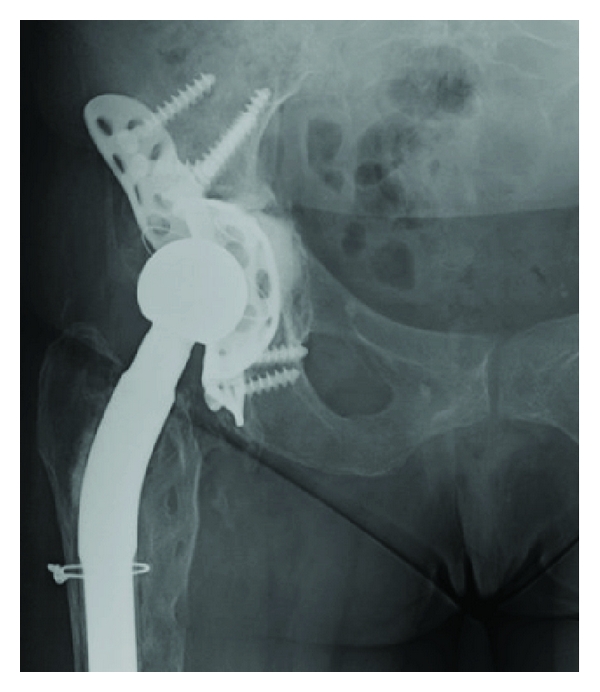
Radiological anteroposterior radiographs of the pelvis showing the Burch Schneider metal cage.
